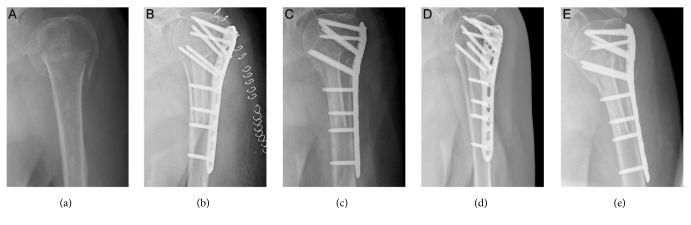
Radiograph of an 80-year-old woman with 4-part PHF treated by locking compression plate with intramedullary fibular allograft. (a) Preoperative X-ray film; (b) postoperative X-ray film; (c) X-ray film 3 months after operation; (d) X-ray film 6 months after operation; (e) X-ray film 18 months after operation.
